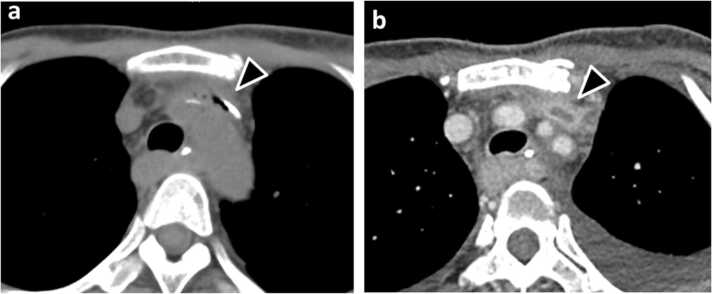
Computed tomography revealing candida thrombophlebitis in the brachiocephalic vein, a)Gas production surrounding a peripherally inserted central catheter b)Residual thrombus after catheter removal.
